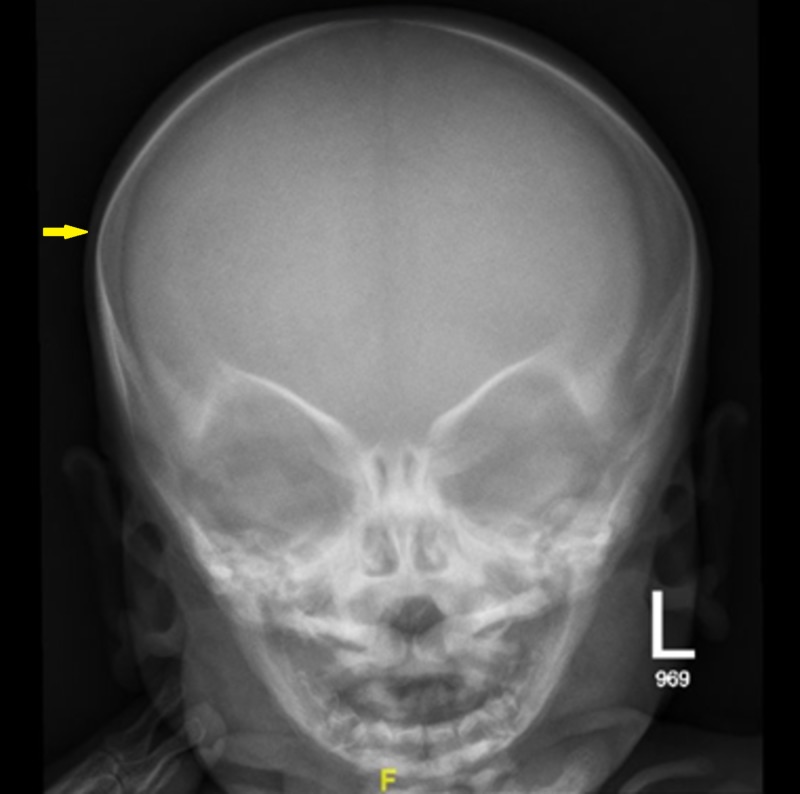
Skull radiograph showed normal scanned osseous structures regarding their density and cortical outline, with no evidence of any fracture line
